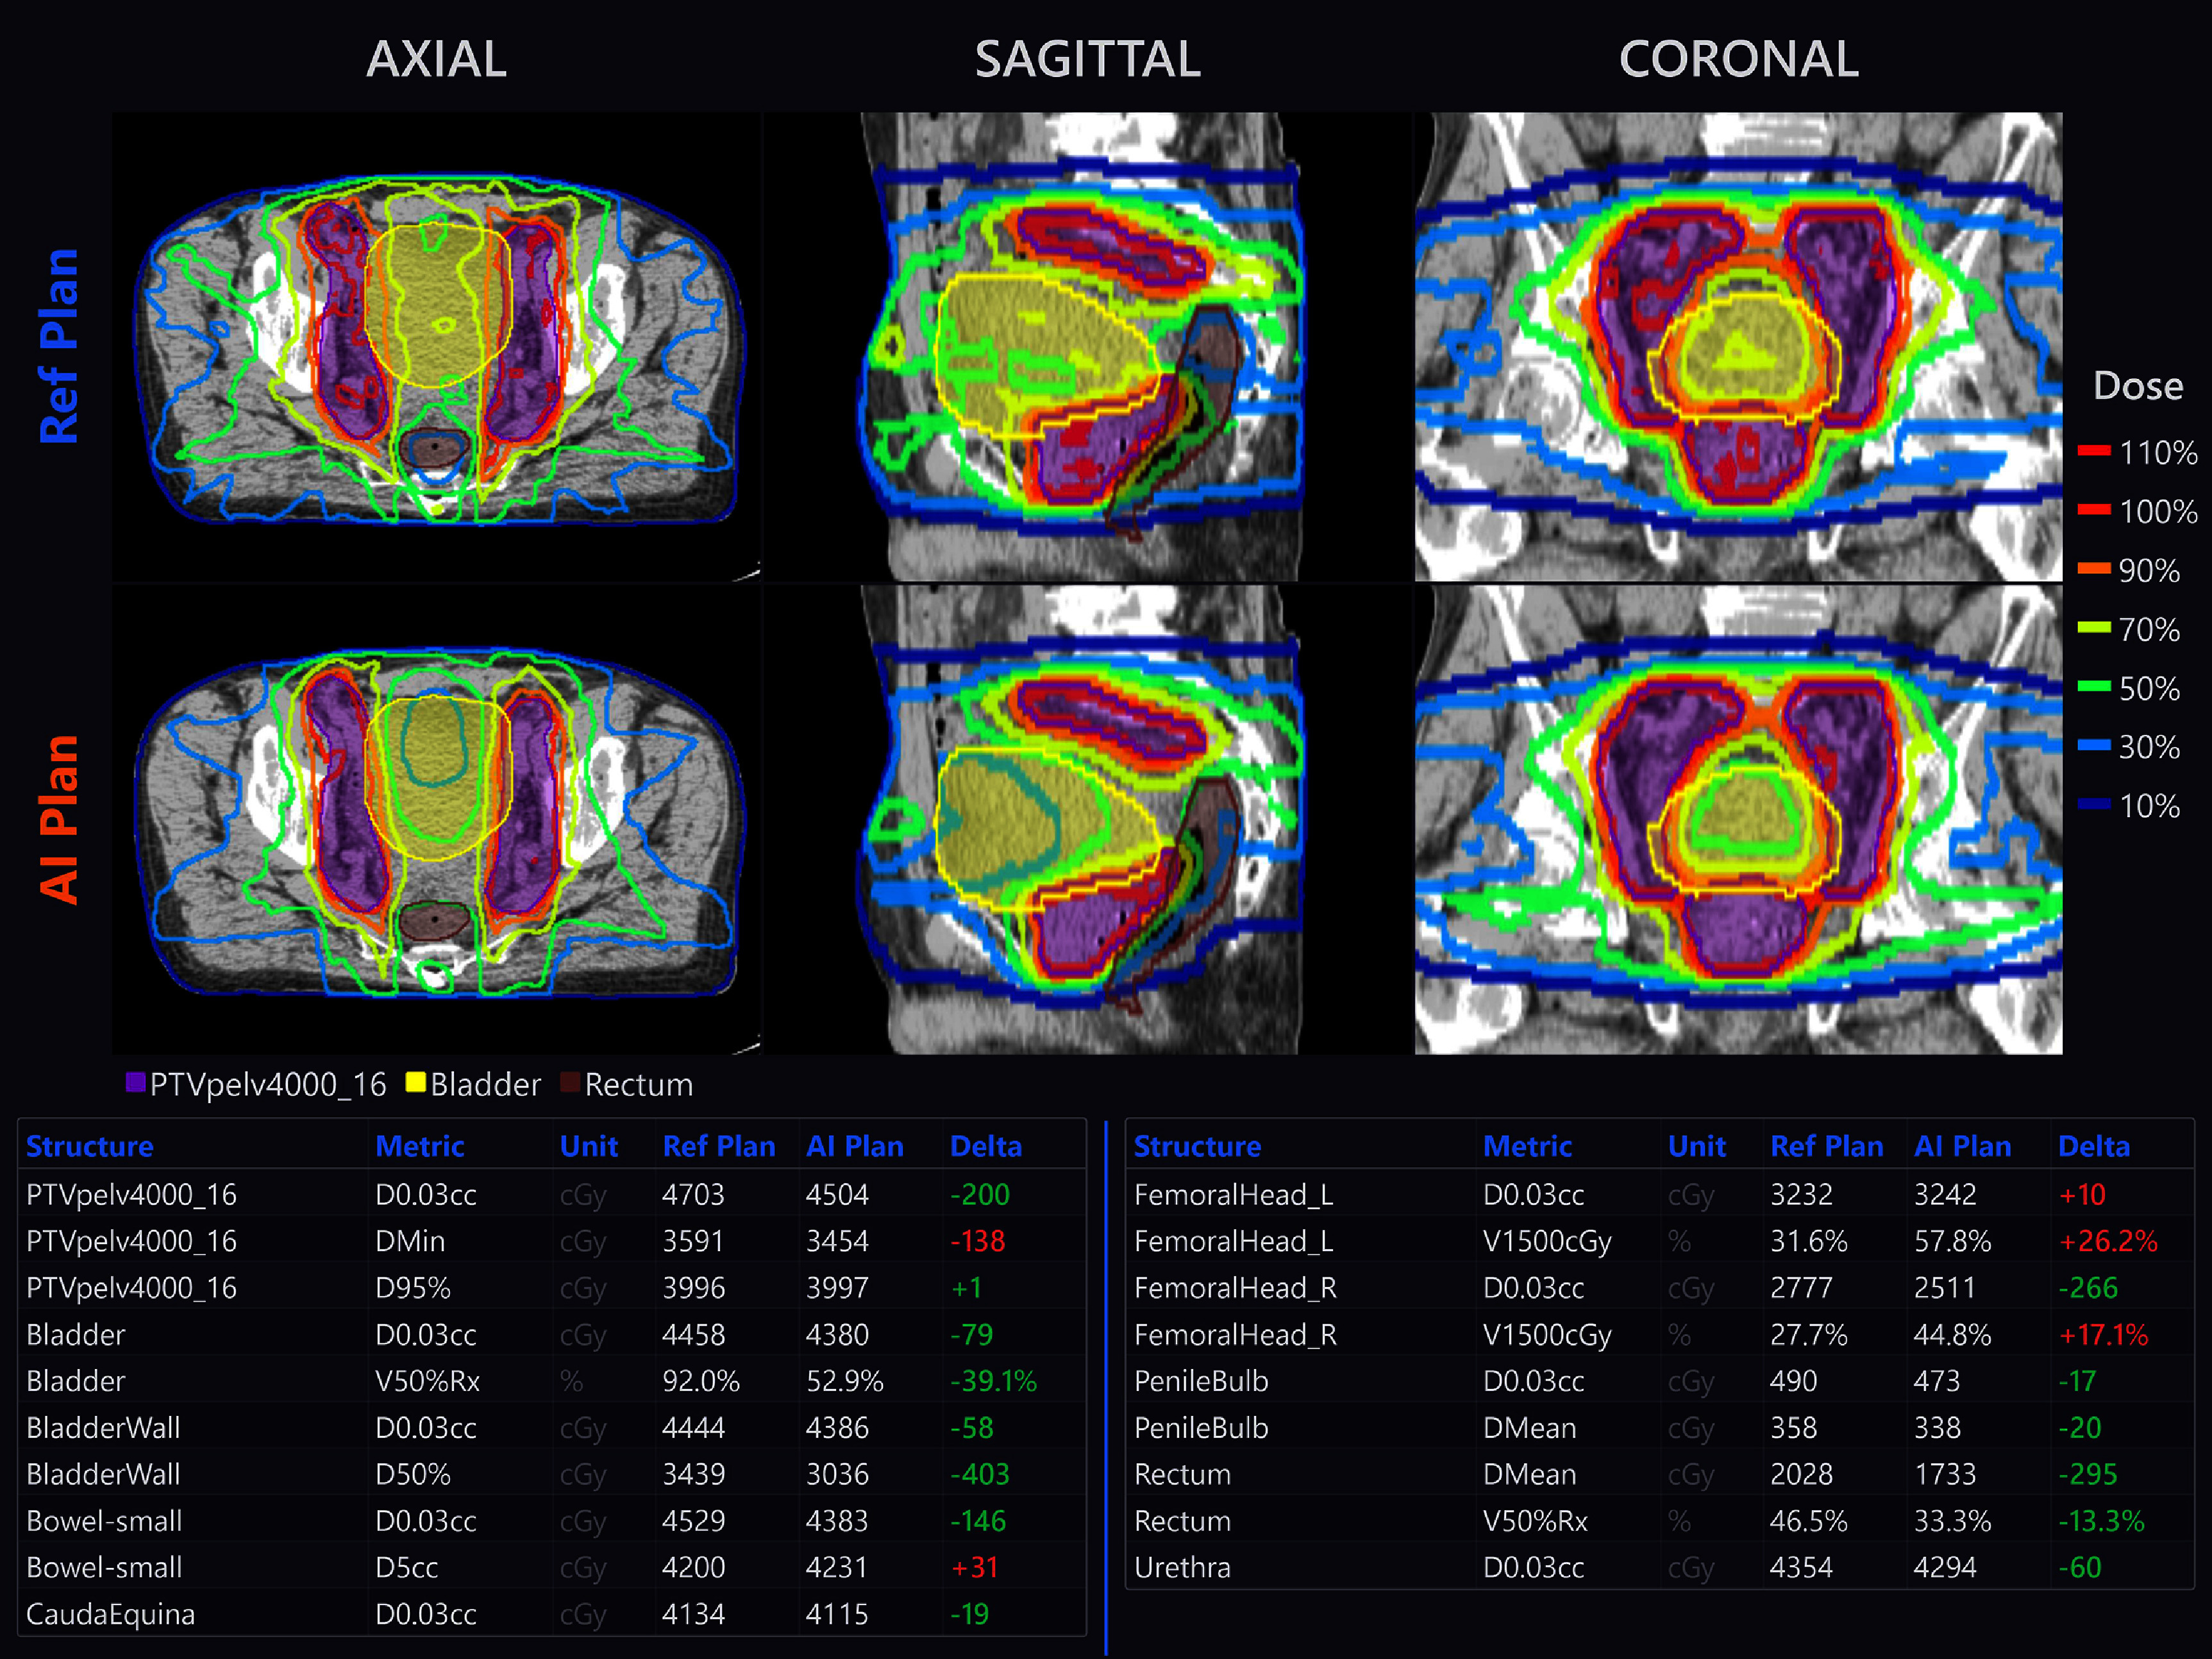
A prostate with nodes case that receives 40 Gy in 16 fractions. Top row: clinical reference plan. Bottom row: artificial intelligence (AI) plan. Dose is overlaid on axial computed tomography (CT) images with the planning target volume (PTV) (purple), bladder (yellow), and rectum (brown) contours displayed. Below the images is a dose-volume histogram (DVH) metric comparison between the two plans. Overall, the AI plan achieved reduced bladder and rectum doses without significant cost to PTV coverage.
